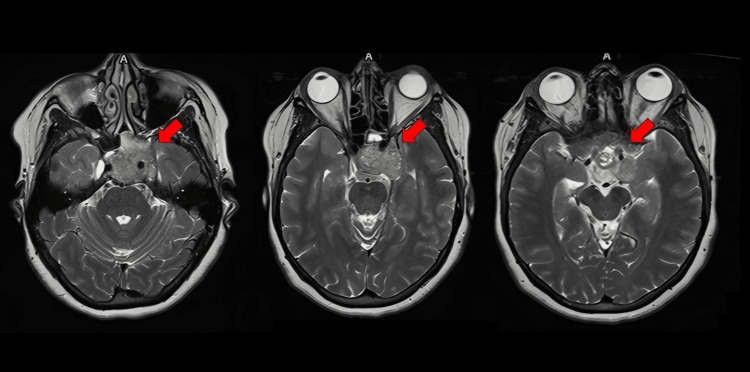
Preoperative axial MRI of the brain showing a large clival mass (red arrows)
